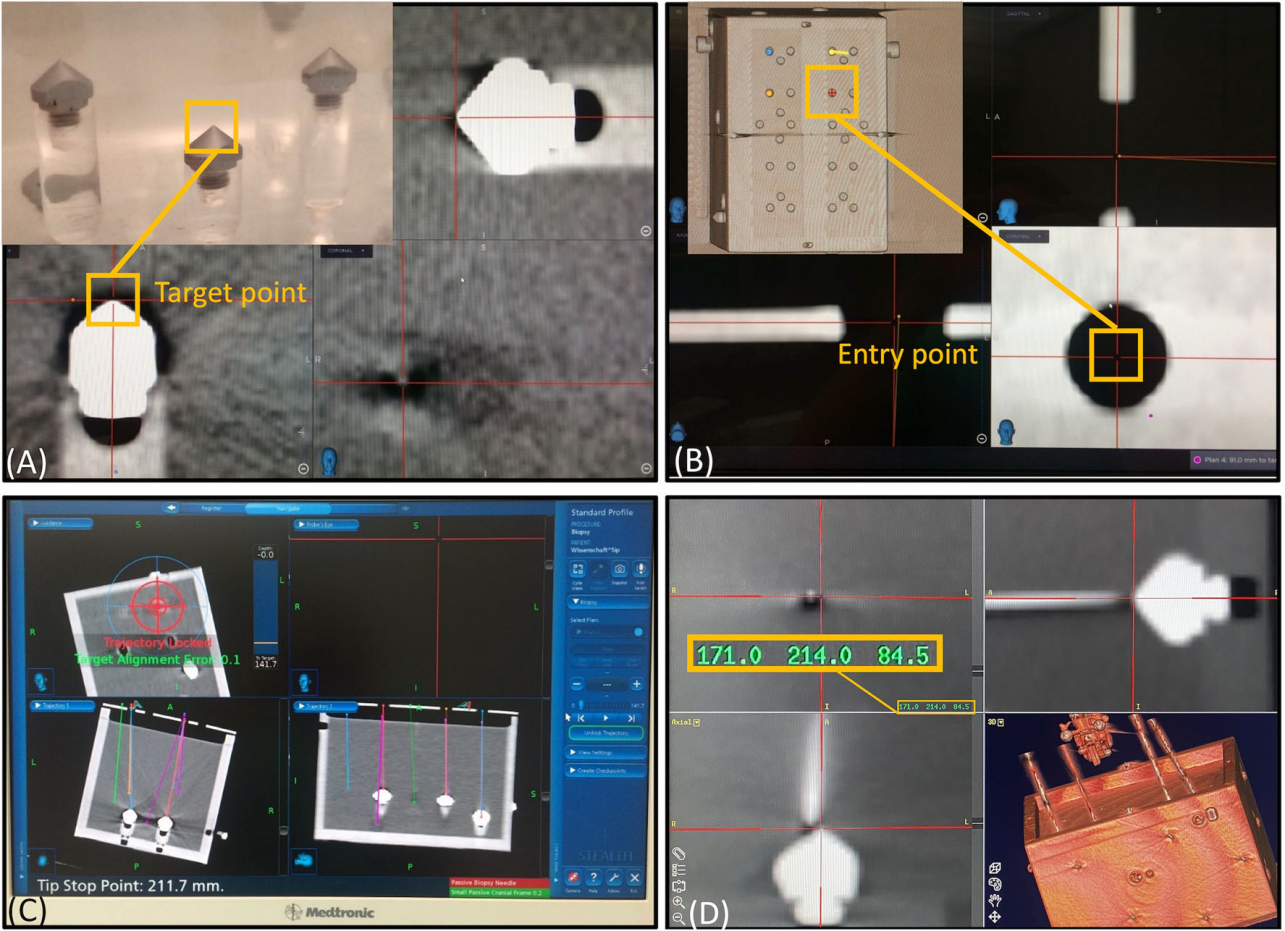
Workstation of the StealthStation S7 (A–C) during the planning and navigation procedure showing the selected target point (A) and entry point (B). After clamping the Vertek probe into the needle guide adapter, the robotic unit is pre-positioned over the targeted trajectory by hand. C The robotic unit is displayed with circles. Fine adjustment is completed when the circles show concentric. D Screenshot of the Treon workstation to evaluate the needle positioning accuracy with the control CT-data. The *x*, *y*, and *z* coordinates (highlighted in the box) were measured by positioning the crosshairs in all three planes. The data was then transferred to an Excel spreadsheet for calculation of the ED and ND
